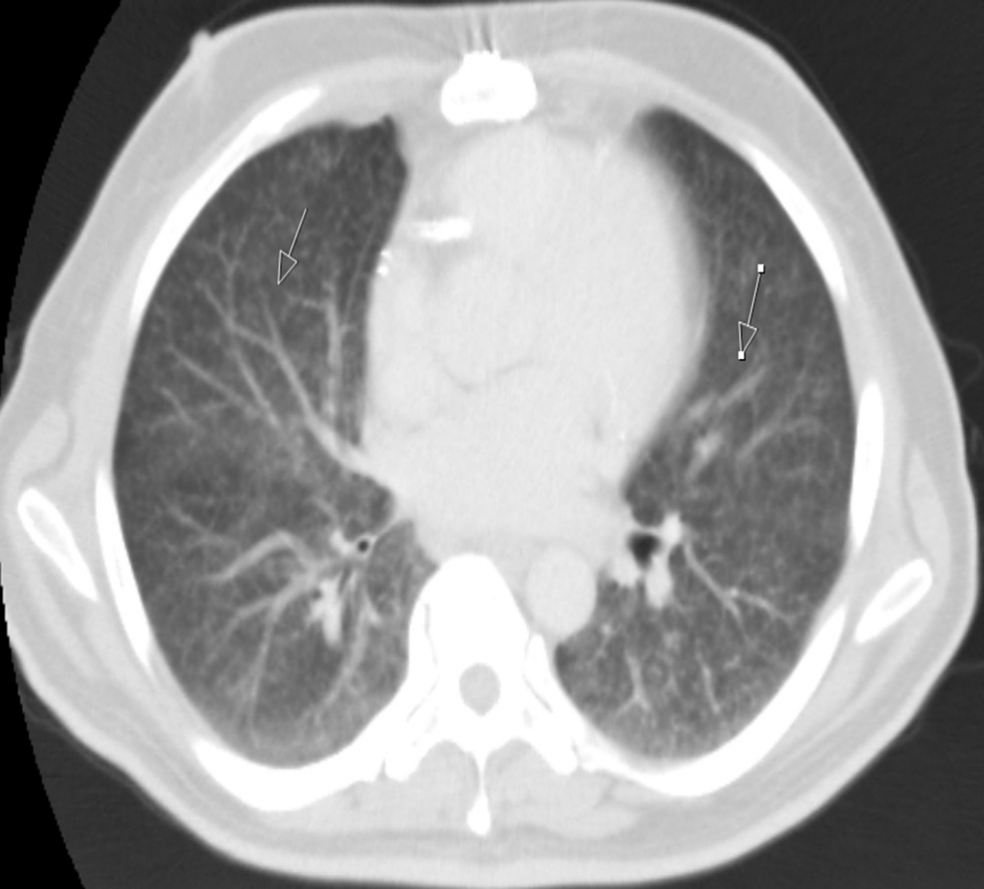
CT CAP showing tree-in-bud appearance in both lungs CAP: chest, abdomen and pelvis
